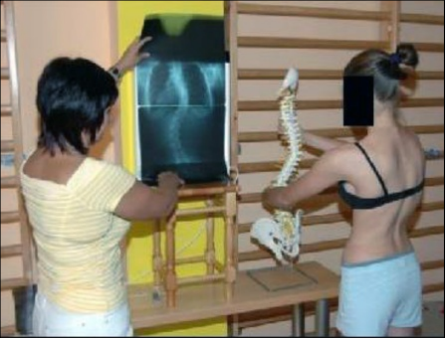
Physical therapist using radiographs and spine models to help the patient visualize and gain awareness of her trunk deformity caused by scoliosis
